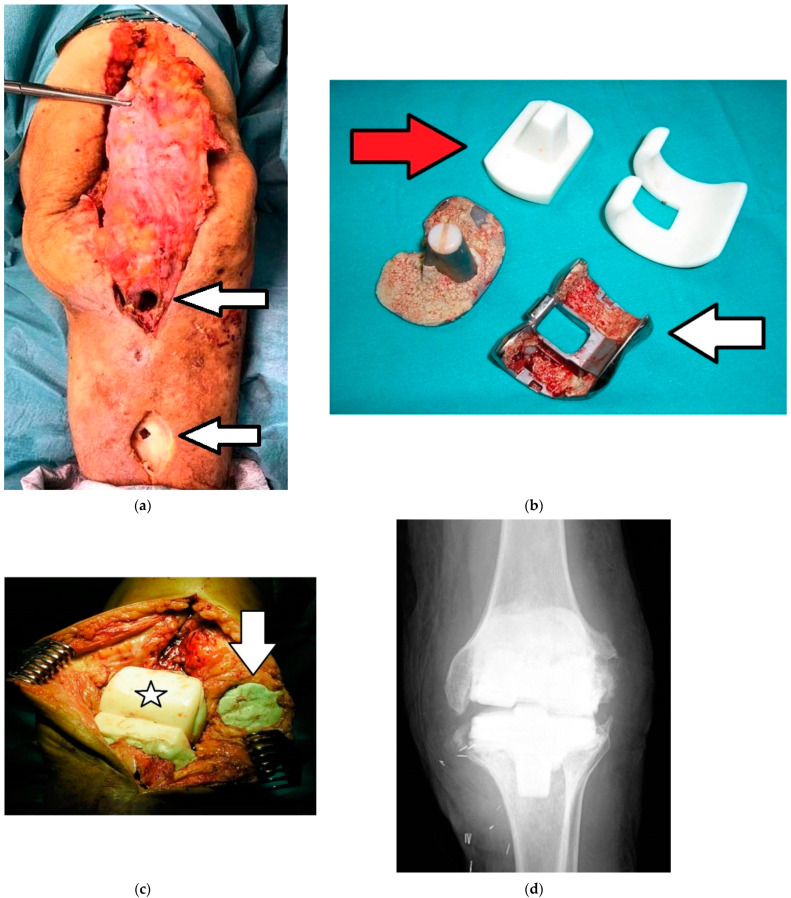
(a–d). Images of the same patient in Figure 1 relative to the first stage of the two-stage revision arthroplasty: (a) Image of the surgical field highlighting two fistulas caused by infection (arrows); (b) Femoral and tibial prosthetic components removed (white arrow) and antibiotic-loaded articulated spacer (red arrow) to implant; (c) Intraoperative image showing the articulated spacer already implanted (asterisk) and bone cement with gentamicin (green color) placed in the patellar component (arrow); (d) Postoperative radiograph showing the articulated spacer implanted.
